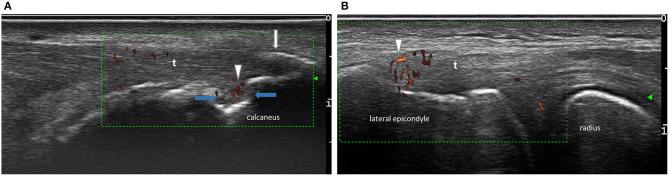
Examples of ultrasound findings. (A) Longitudinal scan of a achilles tendon revealing active PD signal (arrows heads), enthesophyt (white arrow), erosion on insertion (blue arrow), (B) longitudinal scan of tendon insertion on lateral epicondyl with PD signal (arrow heads), t-tendon.
